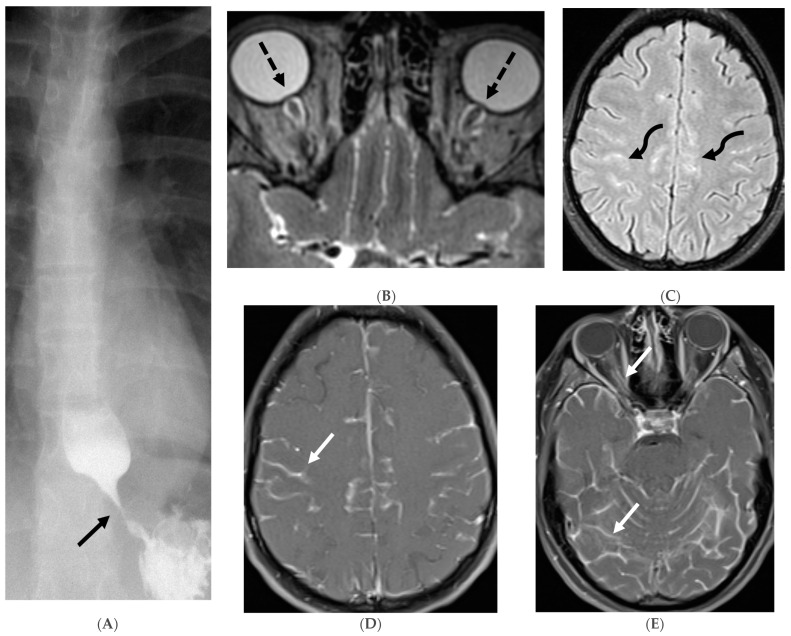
16-year-old female with 4 months history of globus sensation and recent botox injection of lower esophageal junction. Headache and vomiting for past week: Esophagogram (A), Axial T2 orbits (B), axial FLAIR (C) and post contrast T1 (D,E): Narrowing of the Gastroesophageal (GE) junction with beaked configuration and mild distention of the lower esophagus likely from early achalasia (black arrow). There is bilateral papilledema indicating raised ICP (dashed arrows) and sulcal hyperintensity (curved black arrows). Diffuse LME in the supra-and-infratentorial regions and along optic sheaths raising the concern for leptomeningeal carcinomatosis (white arrows). Pathology: Gastric adenocarcinoma metastasis.
